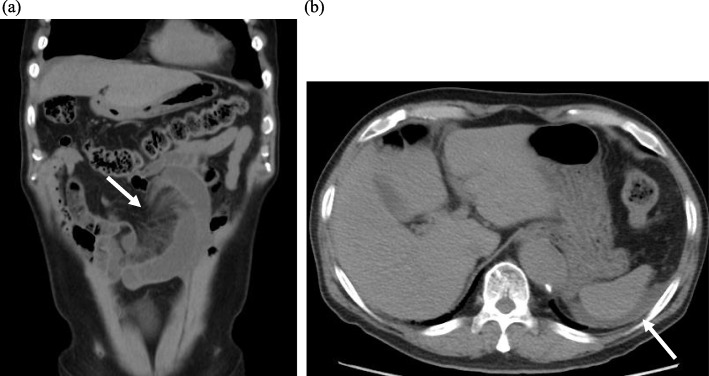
Computed tomography findings. a Computed tomography revealed centralization of the mesenteric vessels and turbidity of the small intestine mesenteric fat around these vessels (arrow). These findings indicated incarceration and ischemic change of the small intestine. b The presence of fluid with high density under the diaphragm (arrow) indicated bloody ascites
